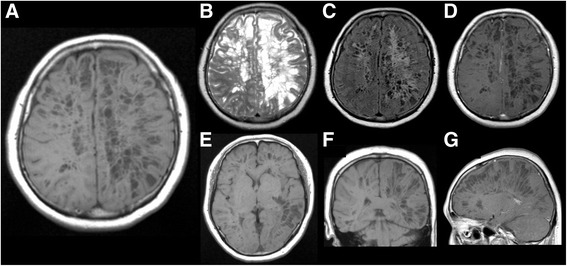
Brain magnetic resonance imaging (MRI) showing multiple, confluent, and oval cystic lesions predominantly in the left cerebral hemisphere on T1 weighted imaging (a, e, f), T2 weighted imaging (b), Fluid Attenuated Inversion Recovery imaging (c), and gadolinium contrast enhancement imaging (d, g). Cystic lesions were found in particular in the angular gyrus and frontotemporal lobes (a, e). They are isointense to CSF (c), surrounded by rim of hyperintensities (c), and observed without gadolinium contrast enhancement (d, g)
